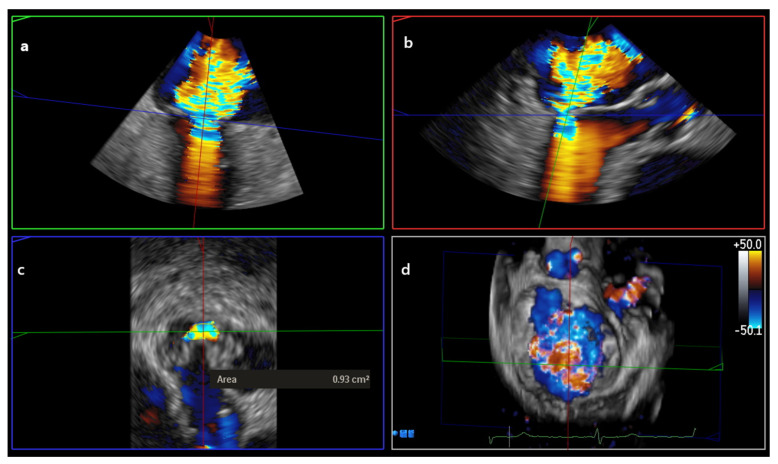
3D VCA measurement derived from MPR of a 3D zoom dataset with color Doppler of the MV. Orthogonal multiplanar reconstruction planes were interactively aligned to the regurgitant jet to ensure true cross-sectional analysis. (a) Commissural plane. (b) Long-axis plane (c) Short-axis plane positioned perpendicular to the jet at the narrowest flow convergence (vena contracta) level, allowing direct planimetric measurement of the VCA. (d) En face MV view, Abbreviations: 3D = three-dimensional; VCA = vena contracta area; MPR = multiplanar reconstruction; MV = mitral valve.
